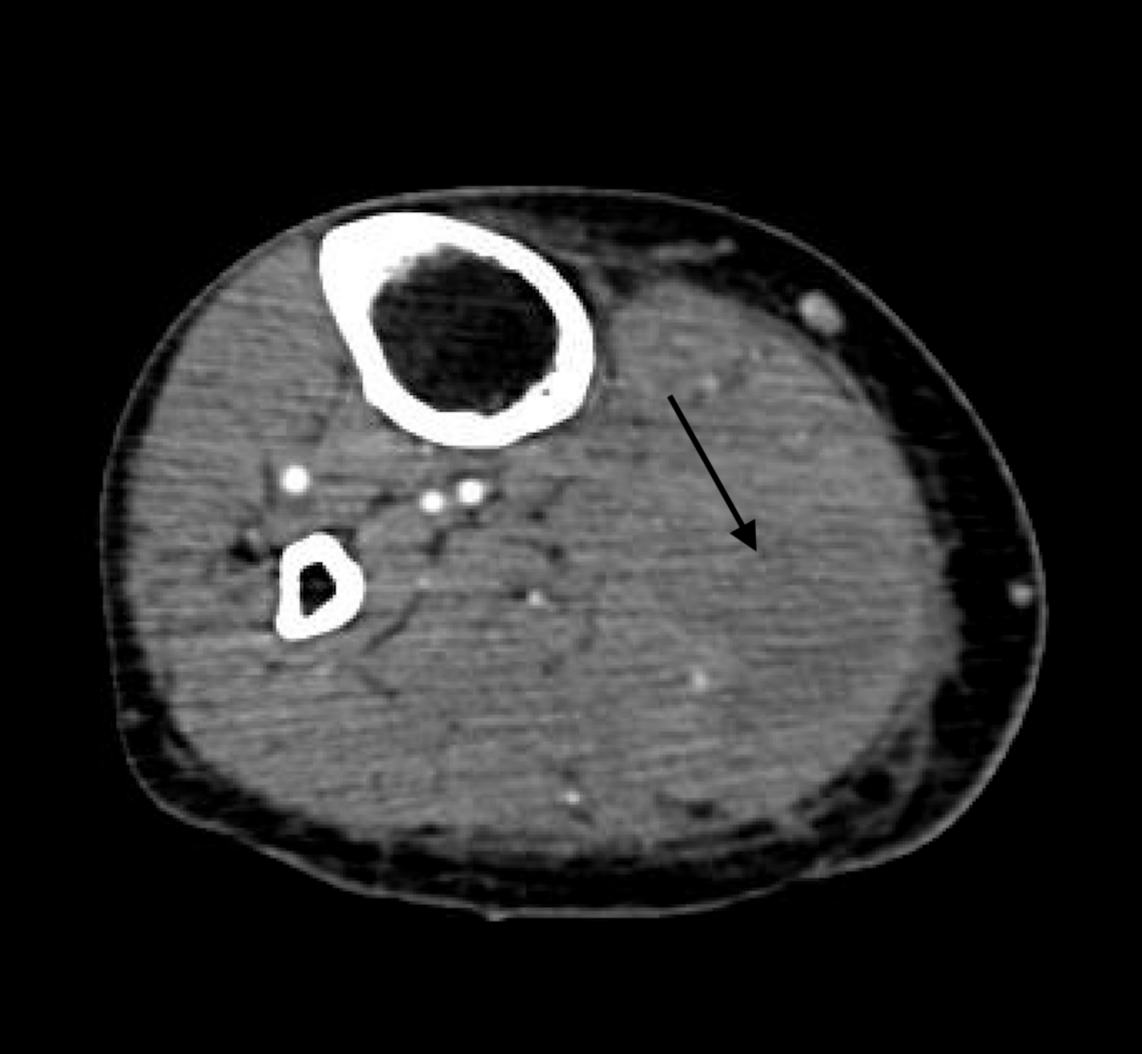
CT angiogram showing fluid collection in gastrocnemius CT: computed tomography
